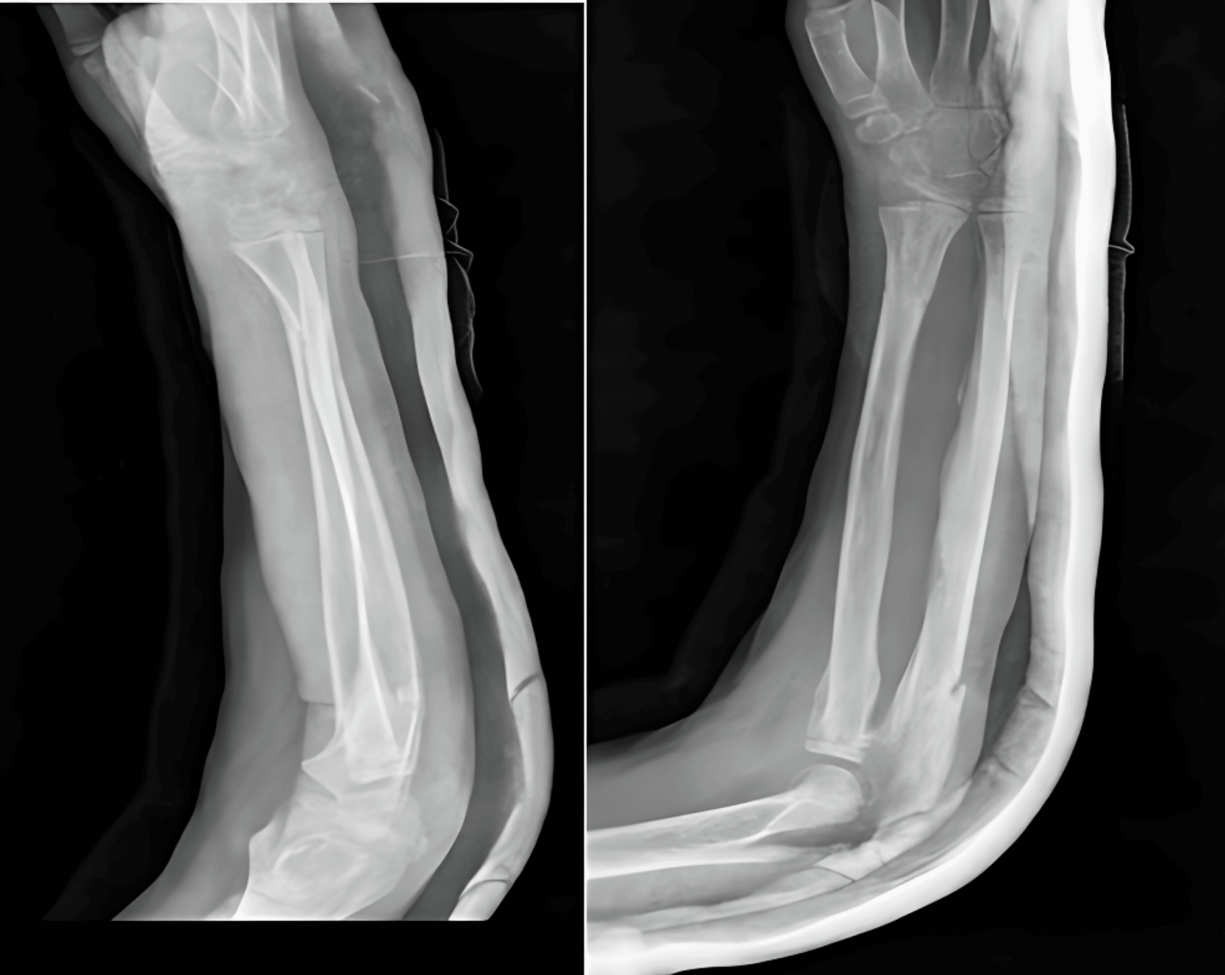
Postoperative X-ray of case 1 after implant removal
